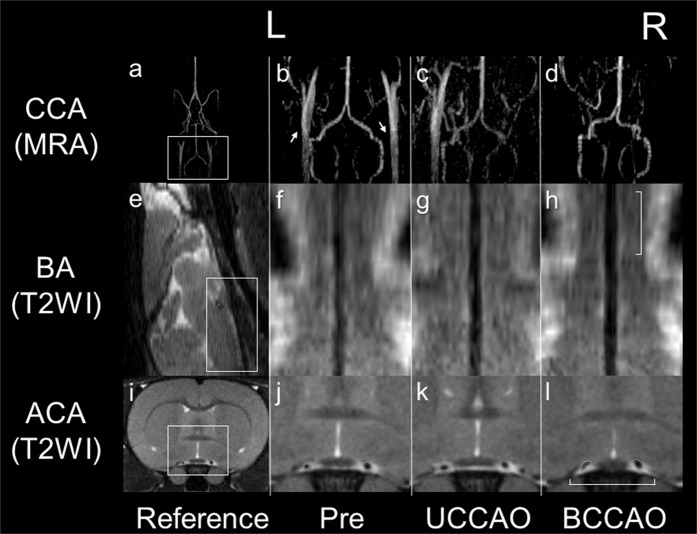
Morphological changes in blood vessels on 11.7 Tesla magnetic resonance (MR) images before and after common carotid artery occlusion (CCAO). The squares in (a,e,i) indicate the references for (b–d,f–h,j–l), respectively. On MR angiography (MRA) images before CCAO (Pre), the CCAs can be identified bilaterally (white arrows in b). L and R are the left and right sides, respectively. After unilateral occlusion of the right CCA (UCCAO), only the left CCA is visualized (c), and after bilateral CCAO (BCCAO), the CCAs cannot be identified (d). The diameters of the basilar artery (BA) measured on curved planar reformation T2-weighted images (T2WI) increase over the occlusion period (f–h). The cross-sectional area of the anterior cerebral artery (ACA) at the section showing the anterior commissure increases slightly in the UCCAO (k) and BCCAO (l) images compared with the image before the occlusion (j). Scale bars, 5 mm.
